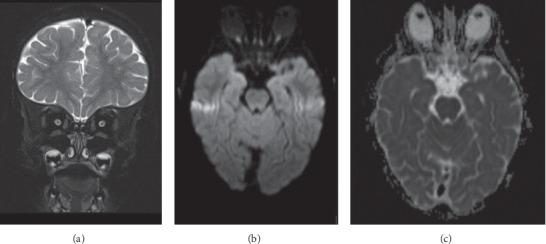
Brain MRI at patient's presentation. Coronal T2 turbo spin echo with fat saturation of the orbits showing normal thickness and T2 signal of the optic nerves. No asymmetry noted (a). Diffusion weighted imaging B1000 (b) and apparent diffusion coefficient map (c). The visualization of the optic nerves is distorted by the presence of artifacts, and allowing for this limitation, no restricted diffusion was observed on the apparent diffusion coefficient map.
